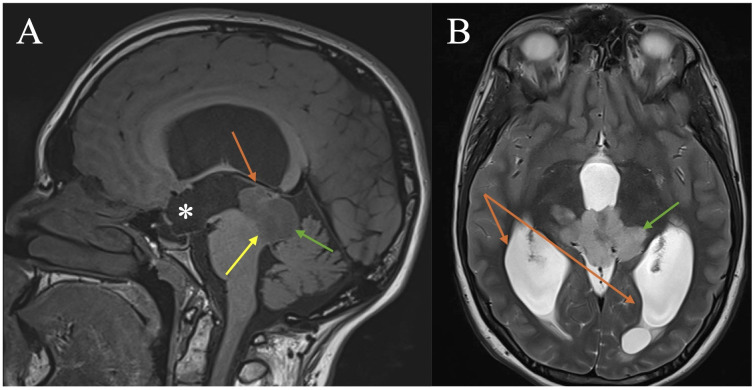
lndex MRI brain depicting tectal plate glioma identified in a 17-year-old female patient. (A) Sagittal MRI T1-weighted image demonstrating a hypointense pineal region mass (green arrow) displacing the pineal gland (orange arrow) superiorly. There is dilatation of the third ventricle with bowing anteriorly of the anterior wall of the third ventricle (lamina terminalis) and displacement of the floor of the third ventricle inferiorly (*). Severe narrowing of the cerebral aqueduct is observed (yellow arrow). (B) Axial MRI T2-weighted image demonstrating a hyperintense lesion centred on the tectum with infiltration into the thalami, right more than left (green arrow). Dilated posterior horn of lateral ventricles at level of pineal region lesion (orange arrow).
